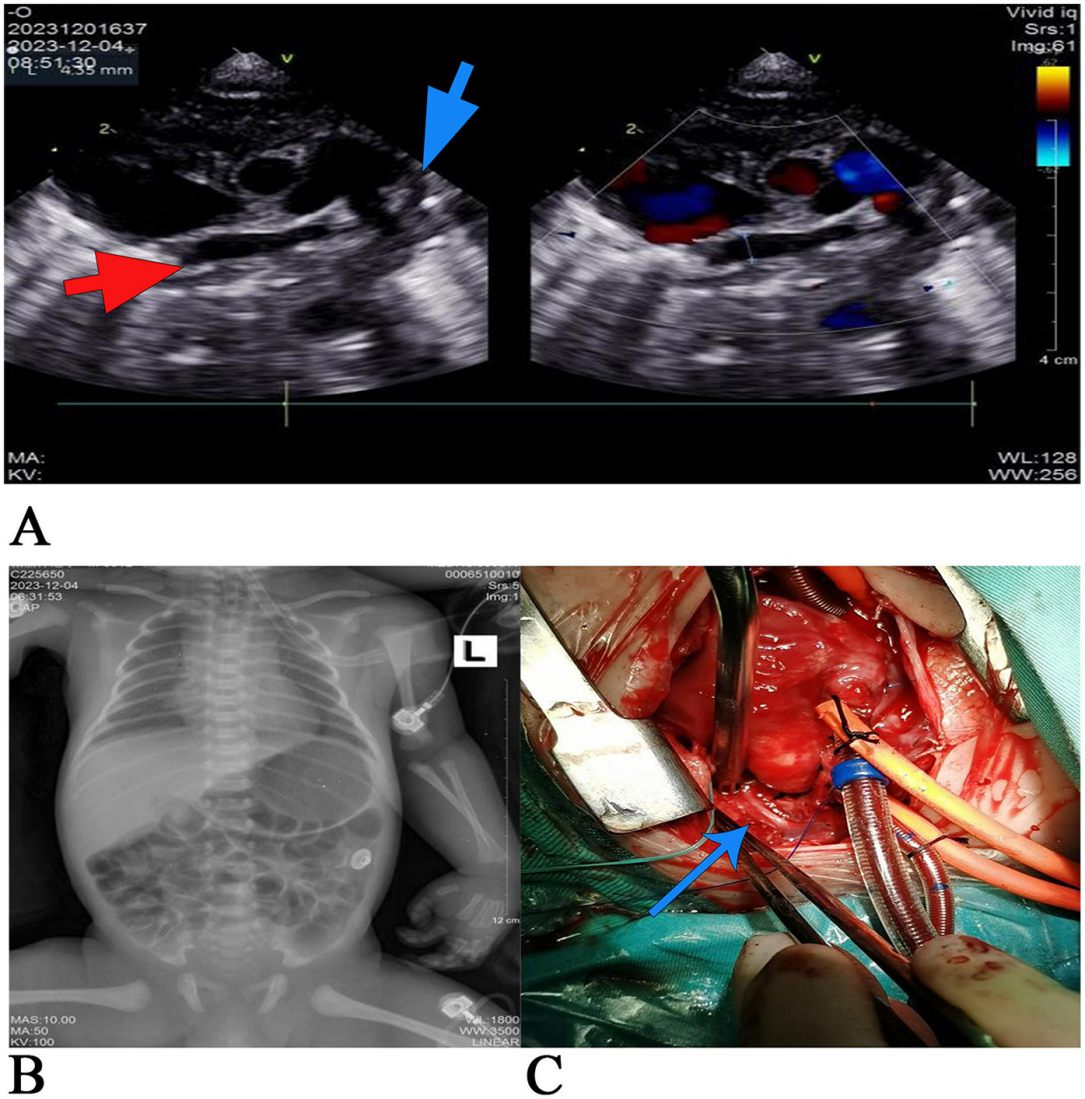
(A) The preoperative two-dimensional echocardiography. The red arrow marks the pulmonary venous confluence, and the blue arrow marks vertical veins. The main pulmonary artery was dilated, none of the four pulmonary veins were connected to the left atrium, and the four pulmonary veins were merged into the common pulmonary vein, which had an internal diameter of approximately 4 mm. Slow blood flow in the vertical veins. The drainage route was not explored and clear (considering: CPVA), the tricuspid valve is not closed, and the left aortic arch can be seen with an open arterial catheter with an internal diameter of approximately 4.5 mm. The venous catheter is open. (B) Chest x-rays taken 18 h after birth showed a slightly enlarged heart shadow, adequate blood flow in both lungs, the tracheal intubation tip at the level of the first thoracic vertebra, a narrow aortic junction, and a flat pulmonary artery segment. (C) Intraoperative image: the blue arrow marks the atretic vertical veins. All four pulmonary veins were not connected to the left atrium and merged into the common pulmonary vein, which joined the left innominate vein through the vertical vein. The vertical vein stenosis and middle and upper muscular atresia were noted.
